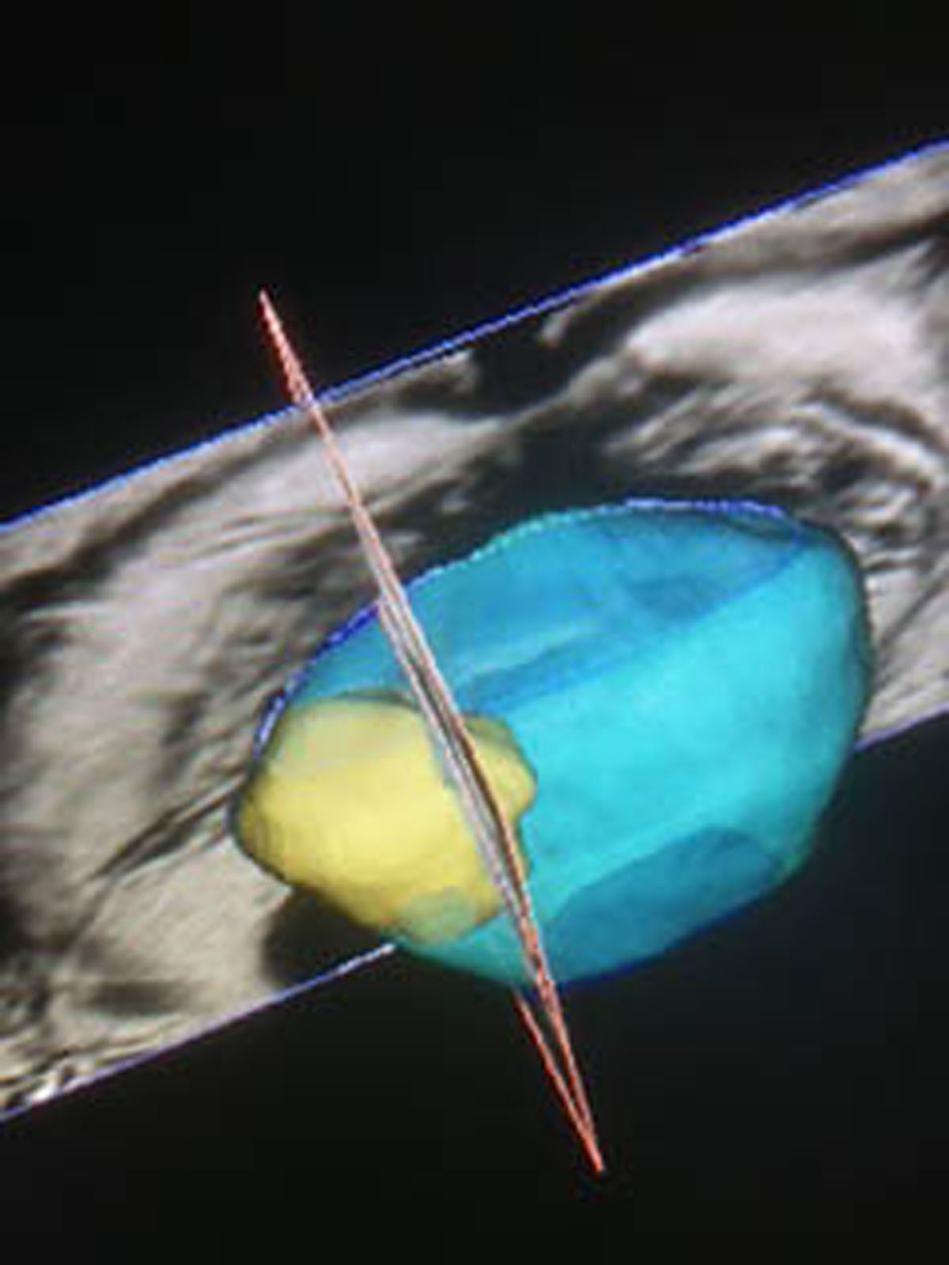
MRI registration with elastic fusion
